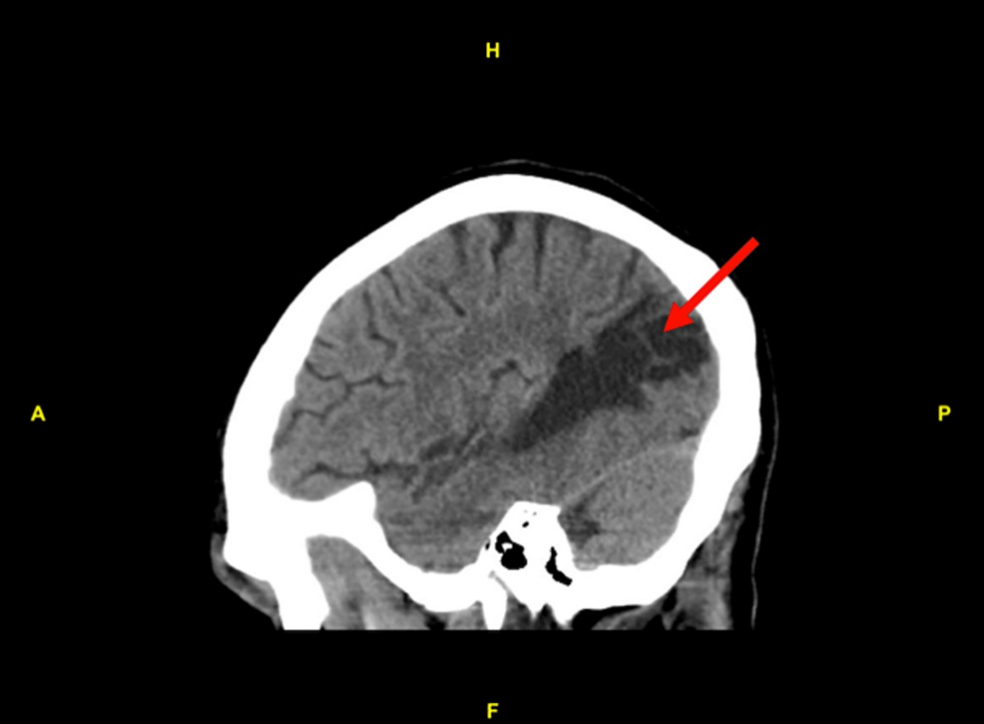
CT head without contrast (sagittal view); re-demonstration of encephalomalacia in the left temporoparietal lobe from the prior infarct
